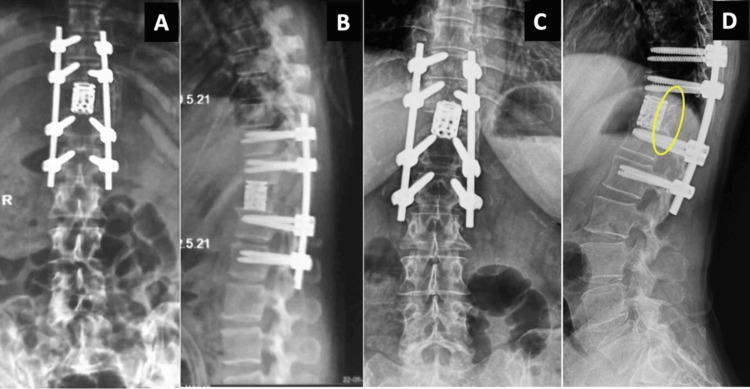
Postoperative radiographs. Immediate postoperative views (A, B) show a pedicle-screw construct spanning two levels above and below, with anterior column reconstruction using an expandable cage. Radiographs at the four-year follow-up (C, D) show that the implant position was well-maintained, as well as nominal cage subsidence and the bridging callus (yellow circle) on the posterior-lateral left side of the cage.
